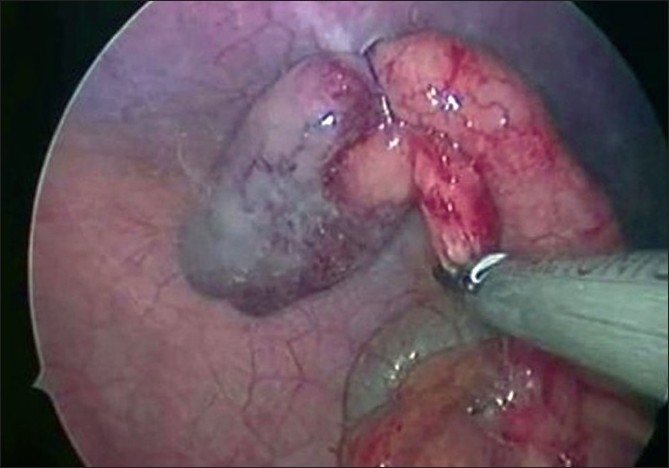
Mesoappendiceal transection with ultrasonic shears.
